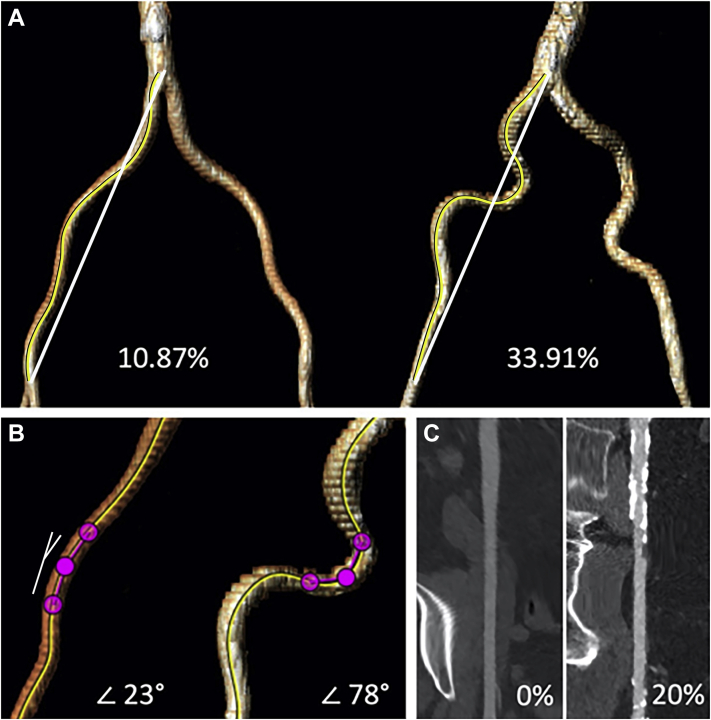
Vascular assessment of the preoperative multidetector computed tomography angiography. (A) Tortuosity index: ([centreline {shown by the yellow line} of flow distance / direct distance {shown by the white line}] – 1) ∗100; an example of a patient with (left) a low tortuosity index and (right) a high tortuosity index. (B) Maximal angulation; measured over the centreline with an arm length of 15 mm; an example of a patient with (left) a low maximal angulation and (right) a high maximal angulation. The measured angle is demonstrated on the left side (white lines). (C) Calcification index; calcium volume and total vessel volume were measured using the Hounsfield unit; an example of a patient with (left) a non-calcified iliofemoral tract and (right) a severely calcified iliofemoral tract.
